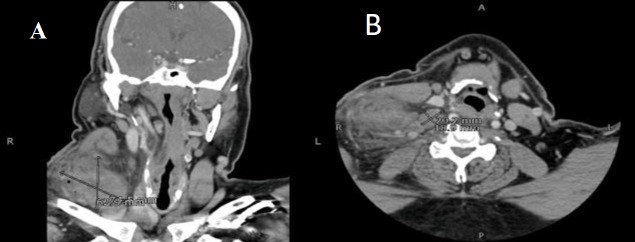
A: CT Scan (Coronal cut) large 7.5 x 6.2 cm mass in the right cervical region Fig-1B: CT Scan (Axial Cut) showing a 2 x 1.9 cm necrotic lymph node along with a mass laterally
